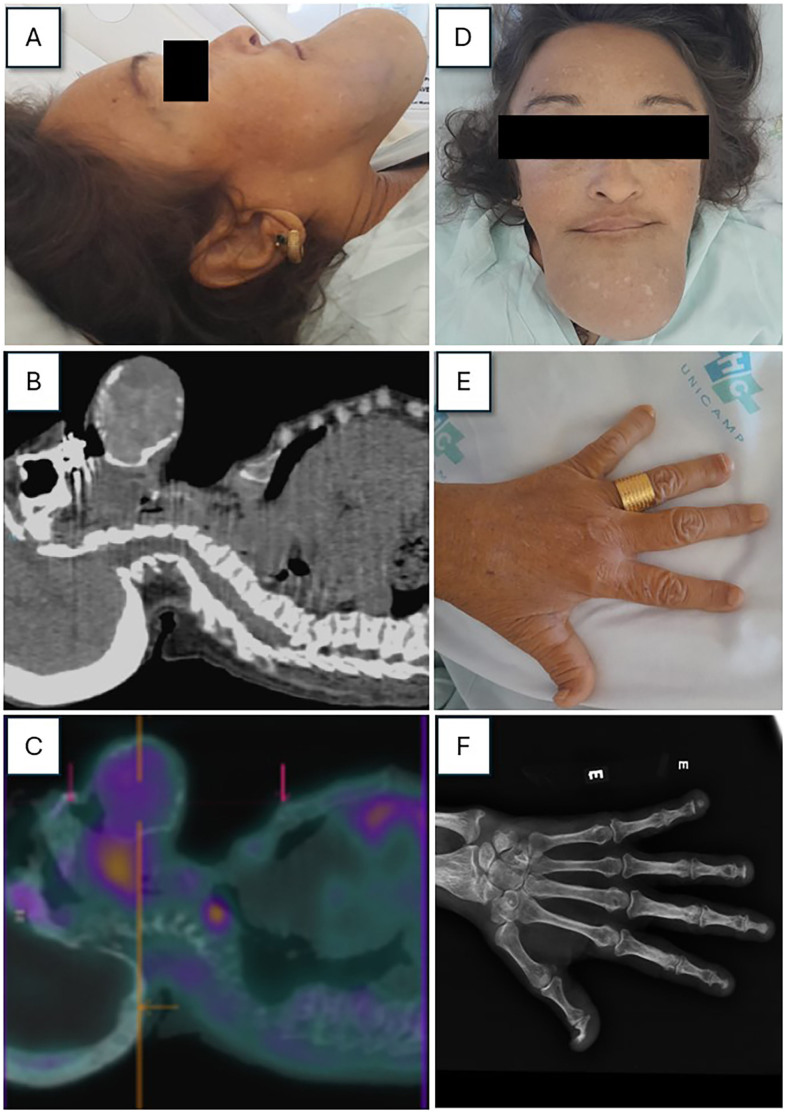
A and D, Giant tumor in the mandibular region, involving the lower dental arch with loss of teeth and bulging and protrusion of the palate; B and C, The 2-hour ^99m^TC-MIBI Spect/CT images show areas of hyper uptake of the radiopharmaceutical in nodular formation located between the esophagus and posterior trachea at C7 level measuring 1.8 cm, compatible with ectopic parathyroid hyperplasia, and expansive heterogeneous bone lesions (brown tumors) in the maxillary sinus, mandibular and mental regions, with disruption of the cortex and involvement of soft tissues in the mandible; E and F, Deformities of the distal phalanges, shortening due to bone resorption, deviations, diffuse bone demineralization, and deformities suggesting osteitis fibrosa.
